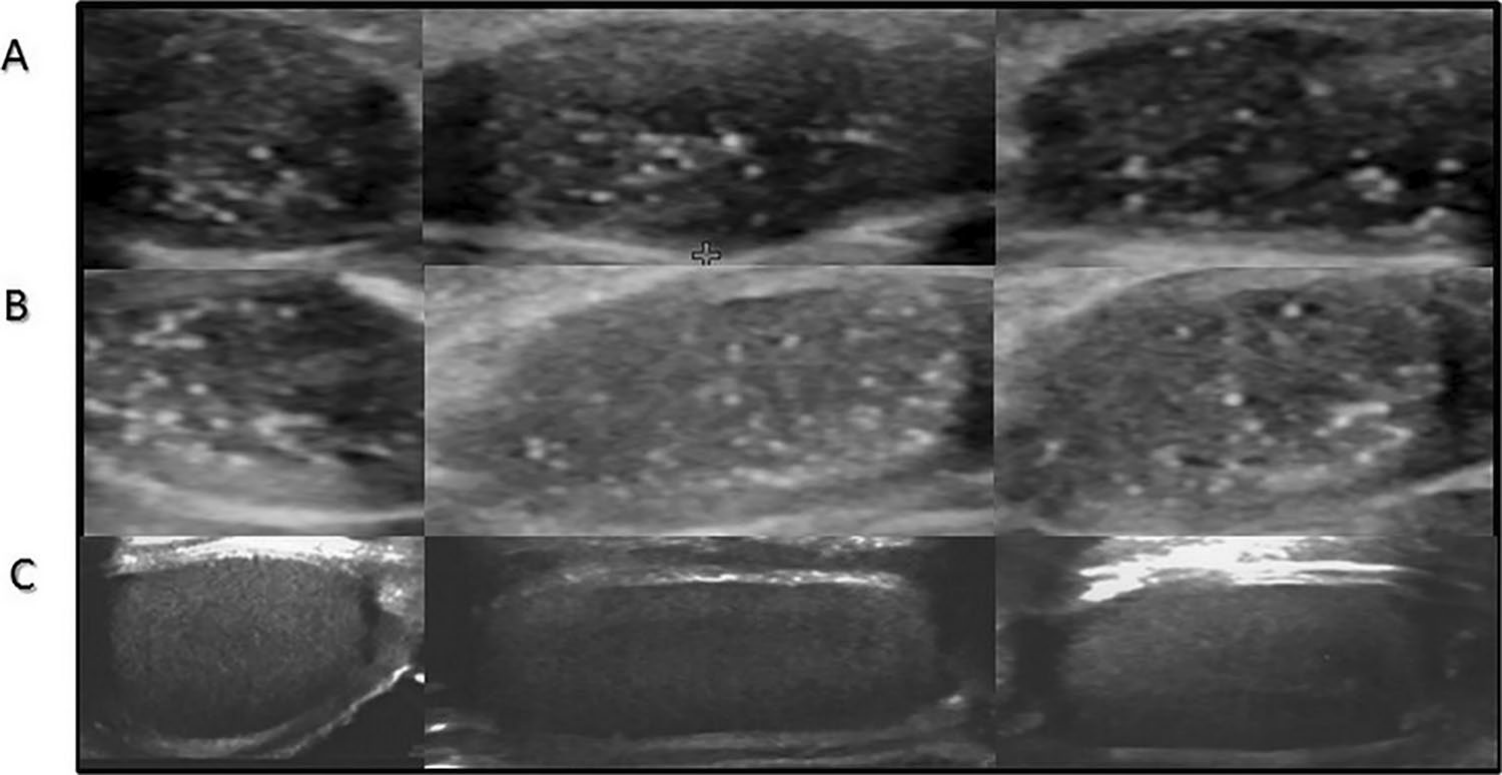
Ultrasound features of testes with TM in patients from prepuberty group. A 7.5 years old, B 1.8 years old, C healthy control 7 years old
